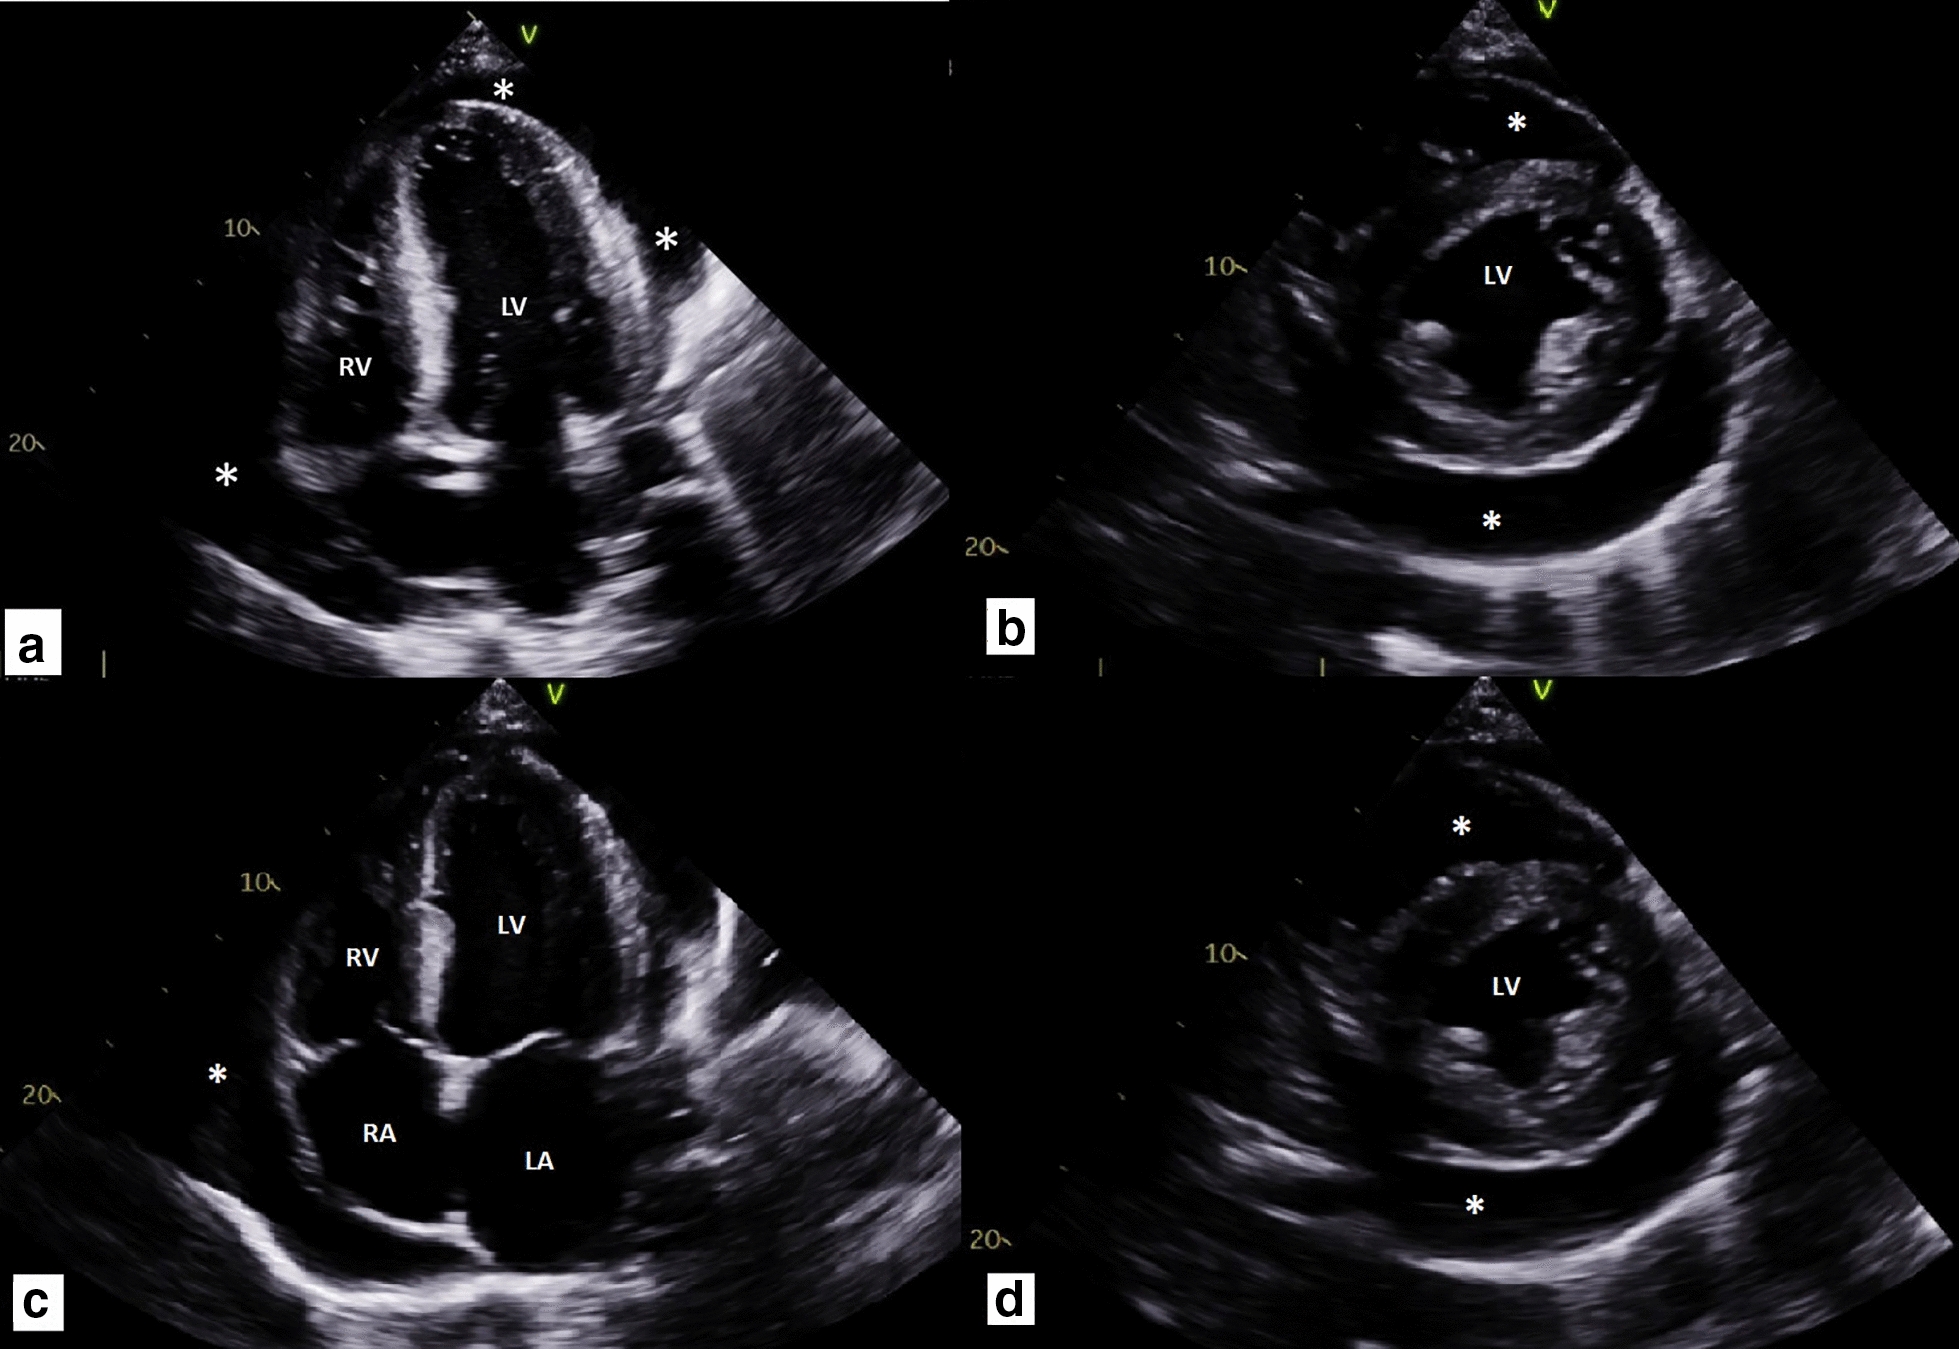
Echocardiogram. Four chamber views in diastole a and systole (c). Short axis views in Diastole b and systole (d). Echocardiography demonstrating a large circumferential pericardial effusion (Asterisks) without hemodynamic compromise, mildly dilated left ventricle (LV) with normal wall thickness, and severe globally depressed systolic function with left ventricular ejection fraction of 10%, normal right ventricle (RV) size and mildly reduced right ventricular systolic function
